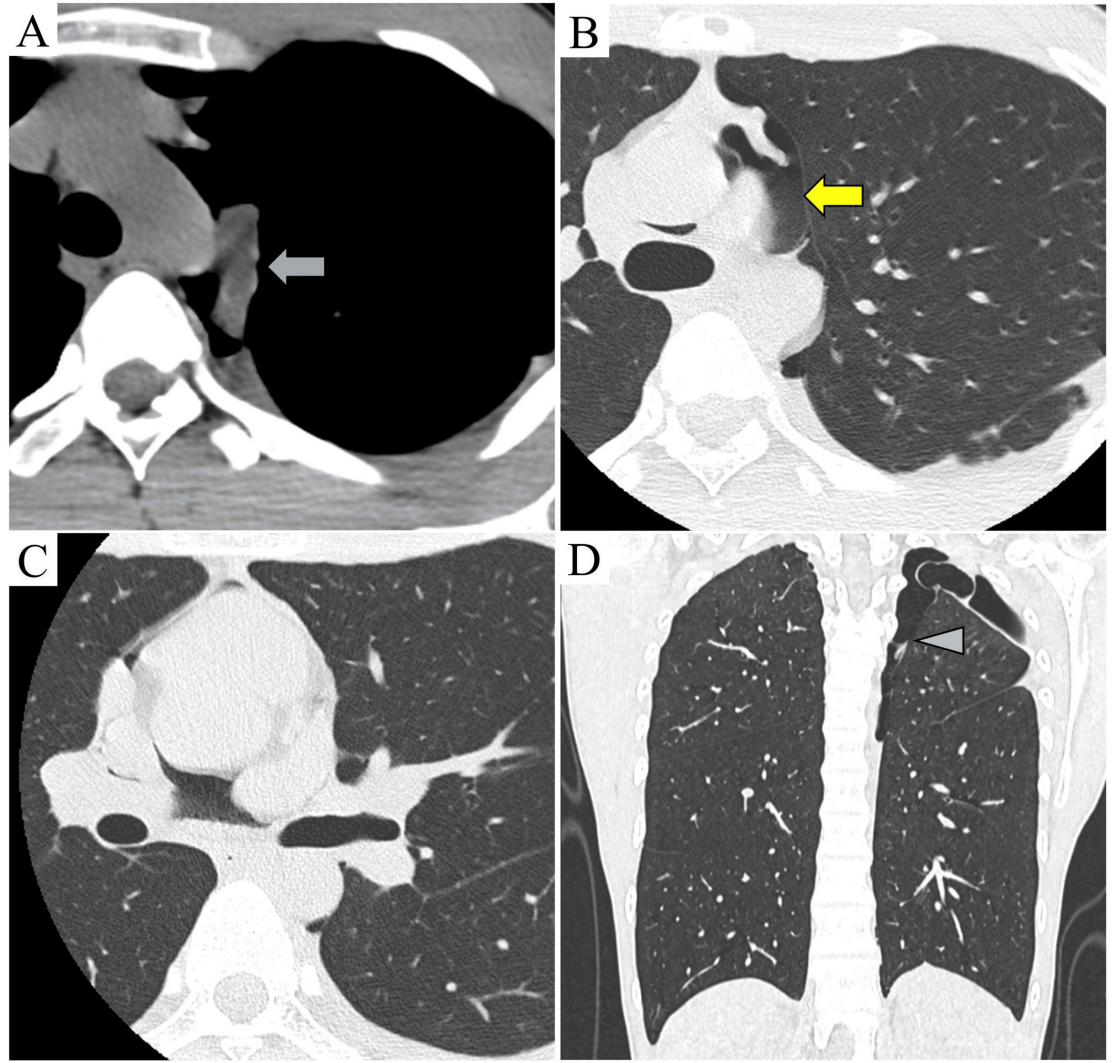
Radiological images. (A) Preoperative plain computed tomography (CT) revealed a 3.2 cm solid mass in the left middle mediastinum (blue arrow). (B, C) Axial CT revealed a pericardial defect (yellow arrow) with pneumopericardium. (D) Coronal CT revealed a left pneumothorax, a bulla in the apical segment of the left upper lobe, and adhesions (arrow head).
